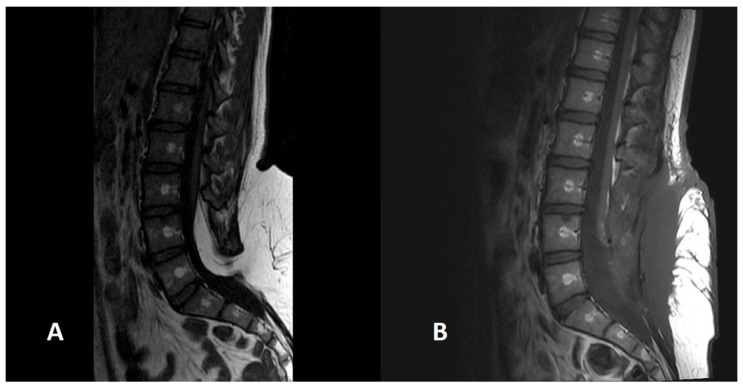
Preoperative (A) and postoperative (B) MR image of a completely removed transitional conus lipoma.
